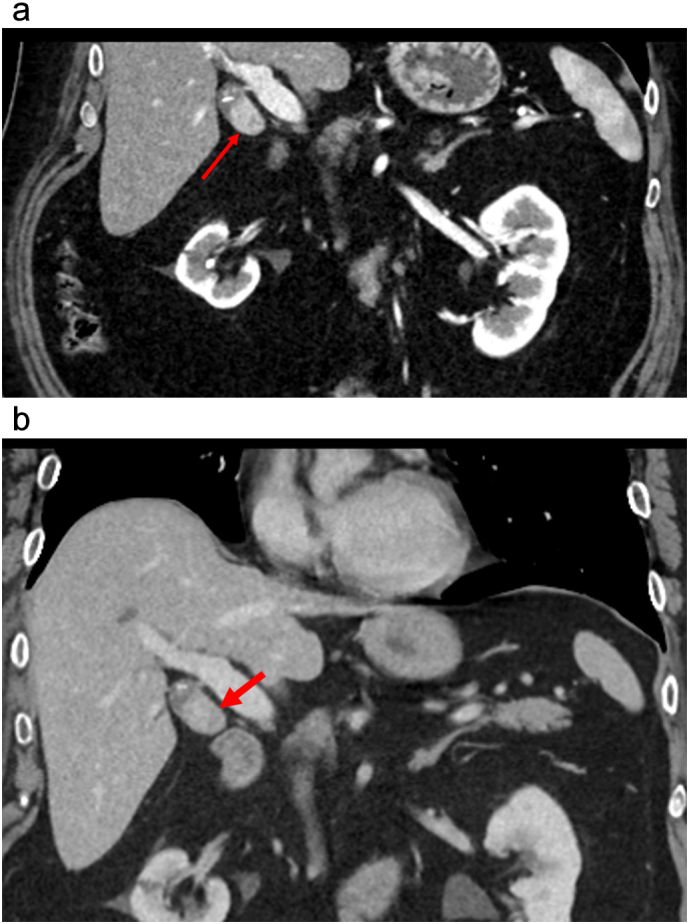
A. Coronal CT arterial phase showing arterial phase enhancing lesion (arrow). B. Coronal CT portal venous phase enhancing lesion (arrow).
